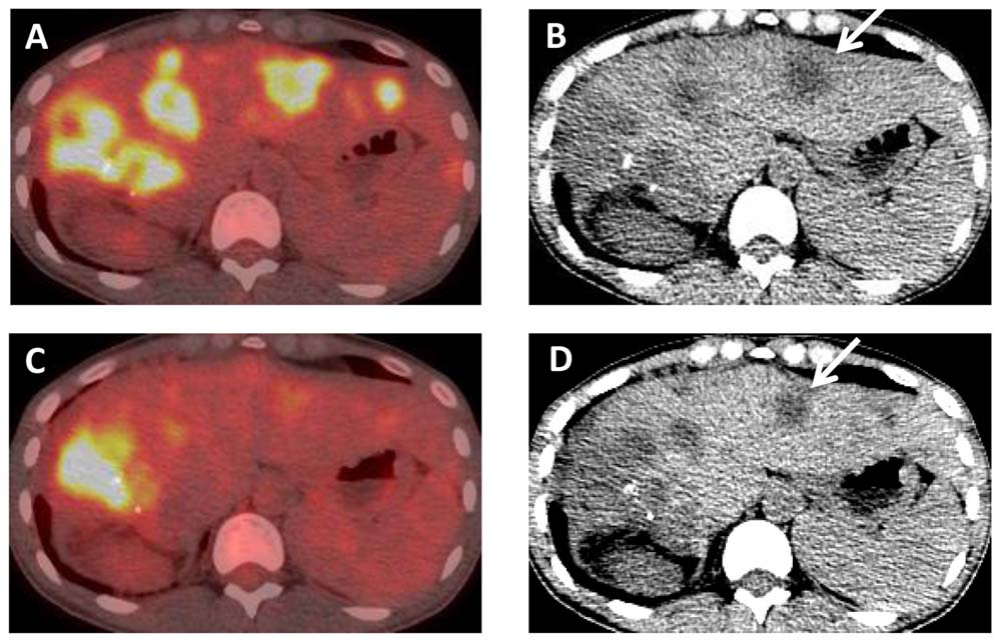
A 37 year old female with *c-MET* amplified CCA, who had progressed on conventional chemotherapy. At baseline, axial (A) fused PET-CT and (B) unenhanced CT images demonstrate multiple FDG avid liver metastases, in this patient who is status post right hepatectomy. After 4 weeks of therapy, (C) axial fused PET-CT and (D) unenhanced CT images show decreased FDG avidity and size of metastases. A representative segment II mass (arrows) has decreased from 2.6 cm to 2.1 cm.
